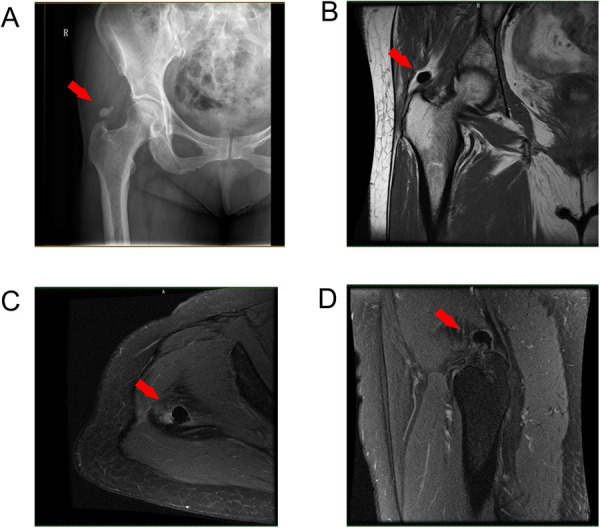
(A) Preoperative plain radiograph, (B) T2 coronal, (C) T1 horizontal, and (D) T1 coronal. View of MRI showing the calcification around the tip of the right greater trochanter (marked with arrows).
